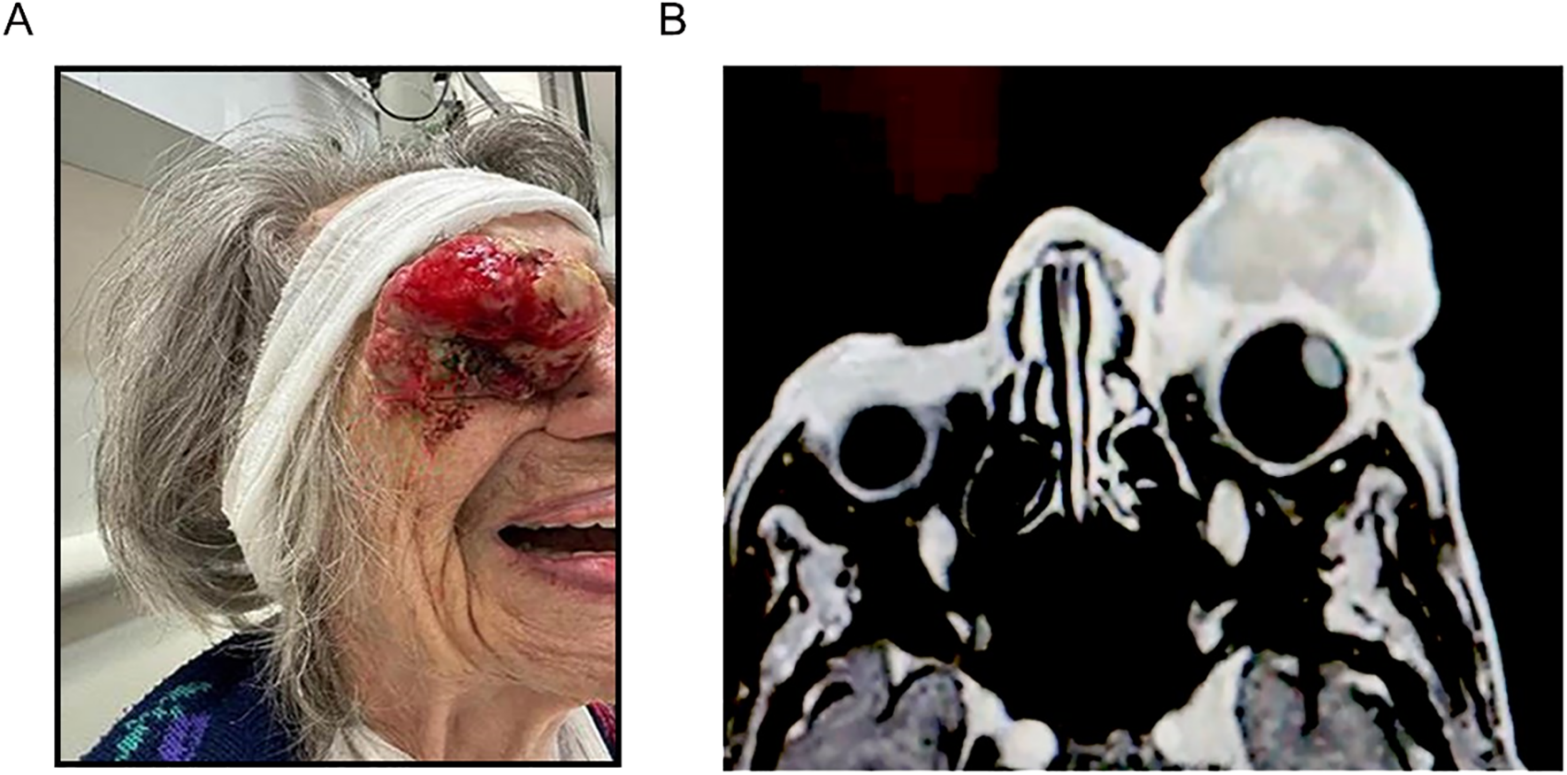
(A) The patient, before receiving treatment, showed a large, exophytic, fungating tumor protruding from her left orbit, known as a conjunctival melanoma. (B) Axial MRI T1 weighted with fat suppression showing the tumor.
